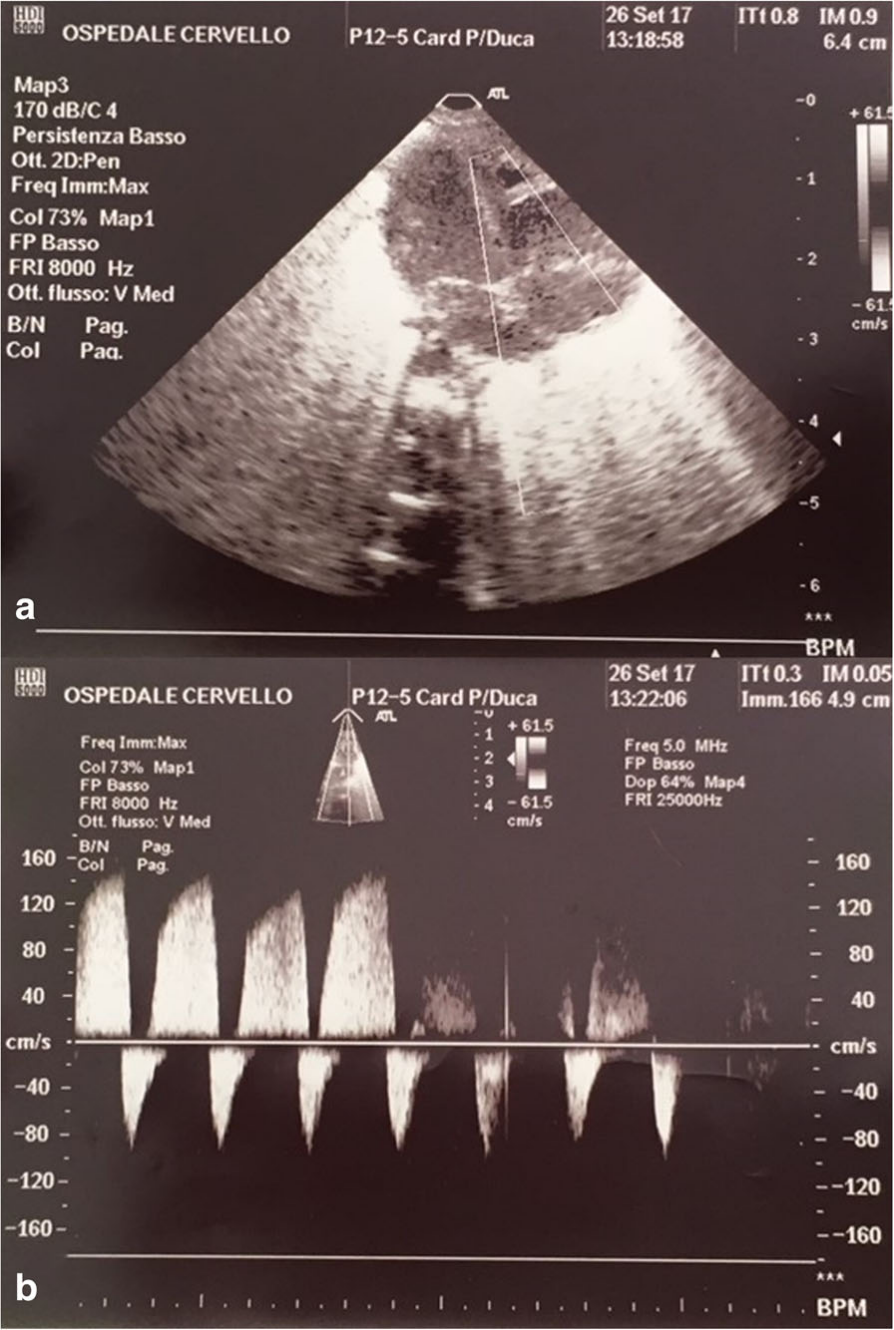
a Echocardiography showing prevalence of right heart chambers. b Doppler ultrasound: sisto-diastolic flow with almost totally left to right shunt
